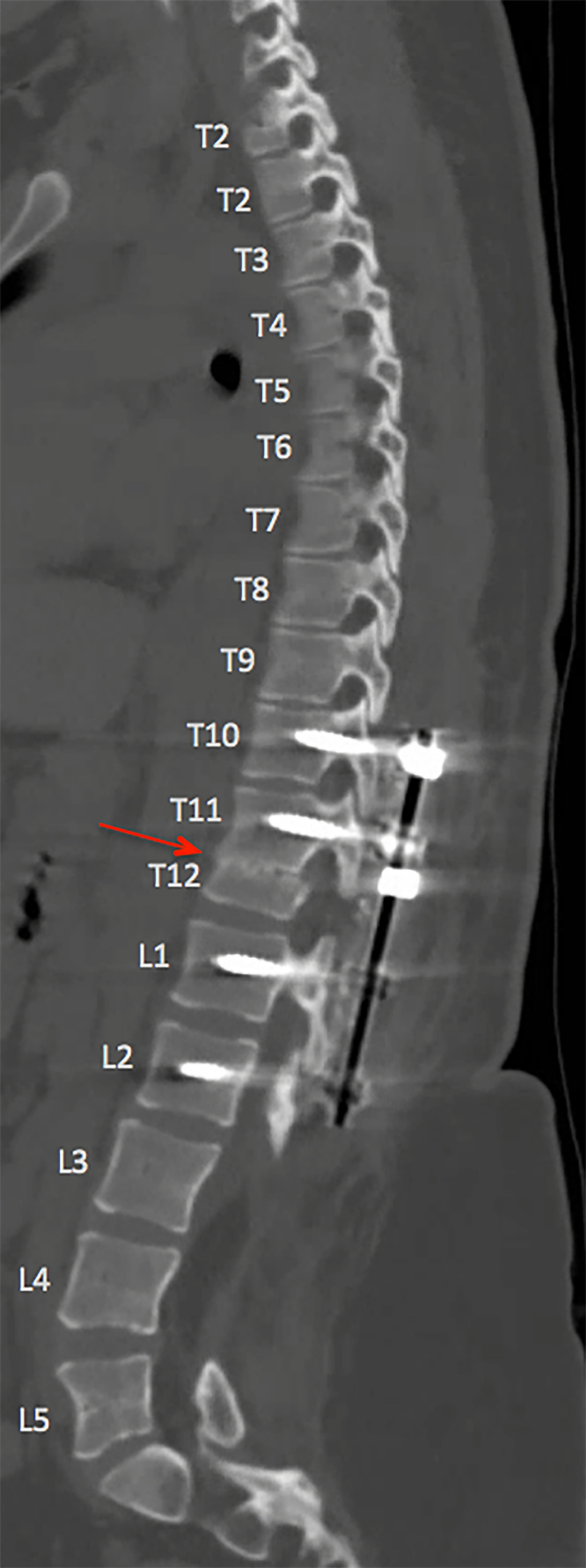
Six-Month Postoperative Sagittal CT Scan Postoperative sagittal computed tomography (CT) scan of the thoracolumbar spine at six months, demonstrating spinal shorting at T12 with posterior instrumented fusion from T10, T11, L1, and L2. Sagittal alignment is maintained with early fusion demonstrated at the vertebral osteotomy site (demarcated by the red arrow).
